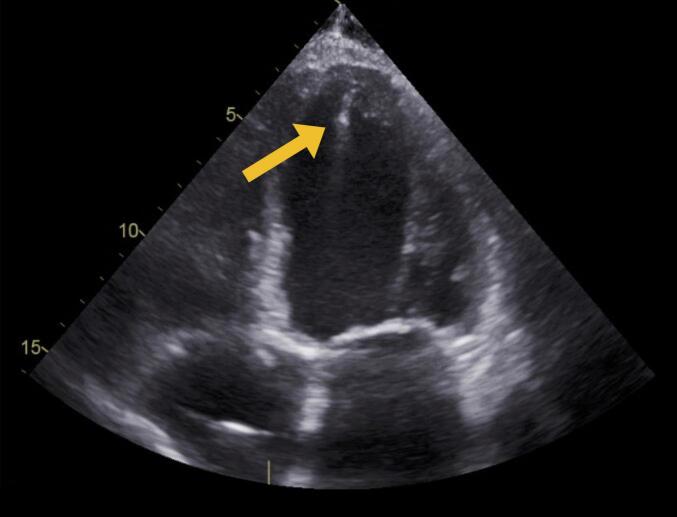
Representative case with apical electrode placement. TTE image obtained from the apical window, demonstrating an apical electrode placement (indicated by arrow). TTE, transthoracic echocardiography.
